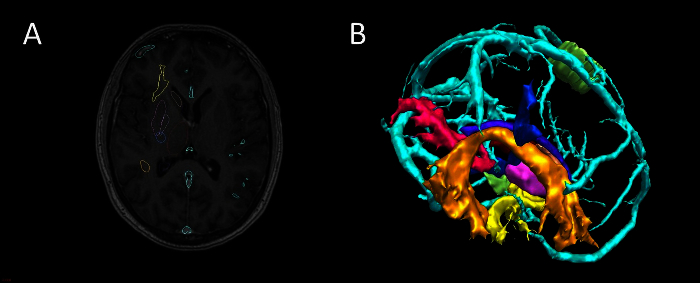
Figure 2. Segmentation and 3D Visualization in In-house Software. (A) axial T1 MRI with superimposed surface models, (B) 3D surface rendering of models (cyan-veins, green- motor hand from transcranial magnetic stimulation, orange- arcuate fasciculus tractography, blue- corticospinal tractography, pink- optic radiation tractography, yellow- uncinate fasciculus tractography, purple- thalamus segmentation). Please click here to view a larger version of this figure.
